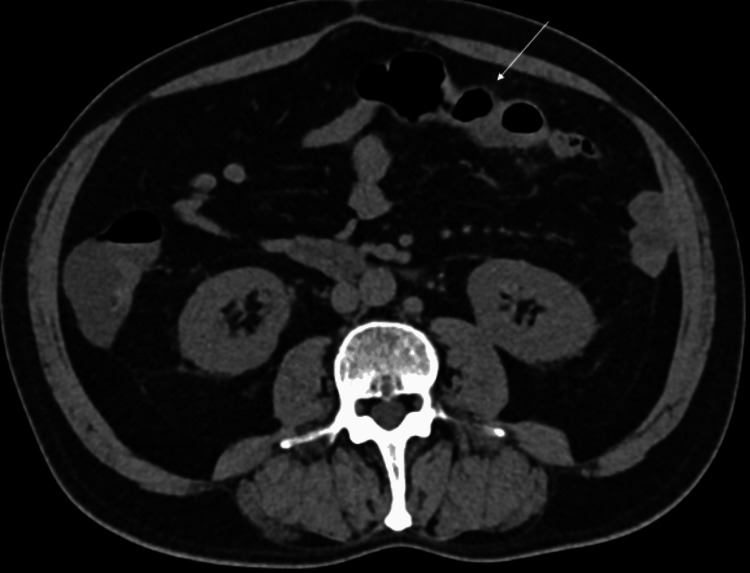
Contrast-enhanced CT of the abdomen and pelvis showing mild thickening of the transverse colon
